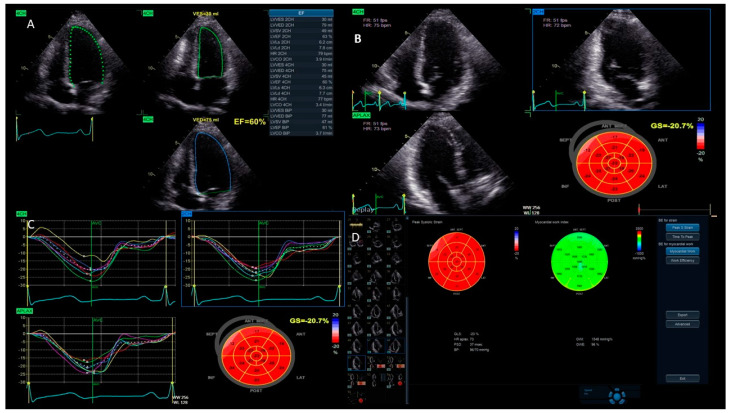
Myocardial strain valuation. Panel (A) shows the auto ejection fraction calculation with a good quality apical acoustic window of the endocardial edges with artificial intelligence technology that allows semi-automatic recognition; in this image, the LVEF = 60%. Panels (B,C) show a 2D left ventricular echocardiography with speckle tracking with a normal global longitudinal strain (GLS) in the range of −21.7%. Panel (D) shows a normal global longitudinal strain (GLS) of −20% and a normal myocardial work index of 1548 mm Hg%.
